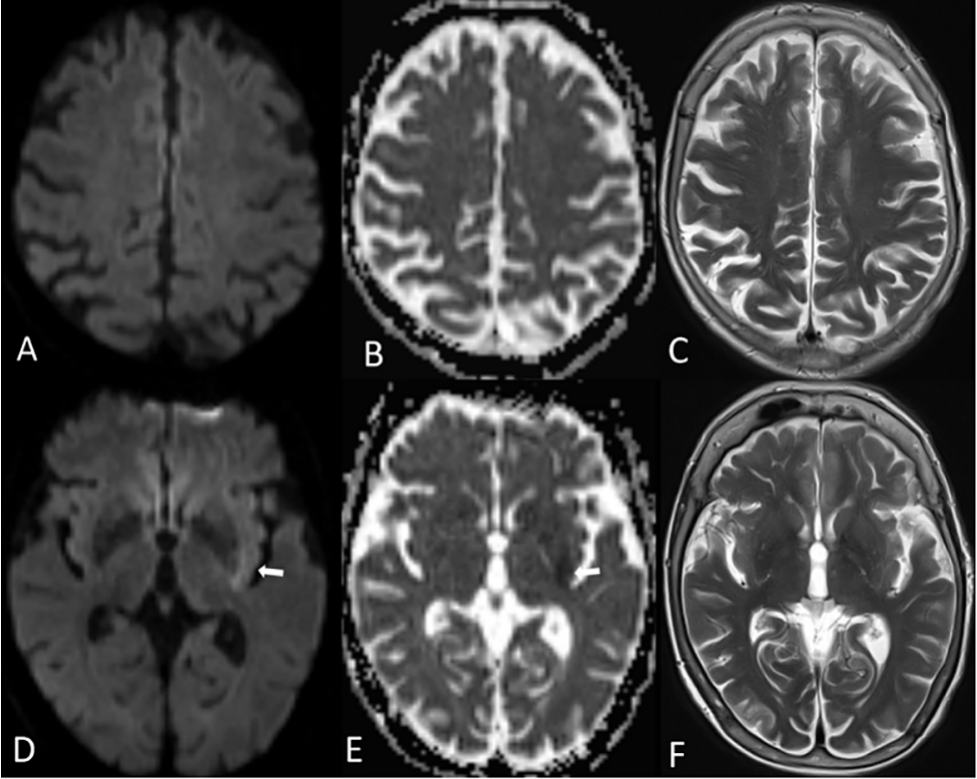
Typical pattern of a well-collateralized patient. 78y old female with left-sided M1-occlusion and a very good pial collateralization (Higashida grade 4); two transverse sections from the acute MRI: DWI (A,D), ADC maps (B,E) and T2w images (C,F). Diffusion restriction was seen in the dorsal part of the lentiform nucleus and the adjacent insula (white arrows in D, E).
